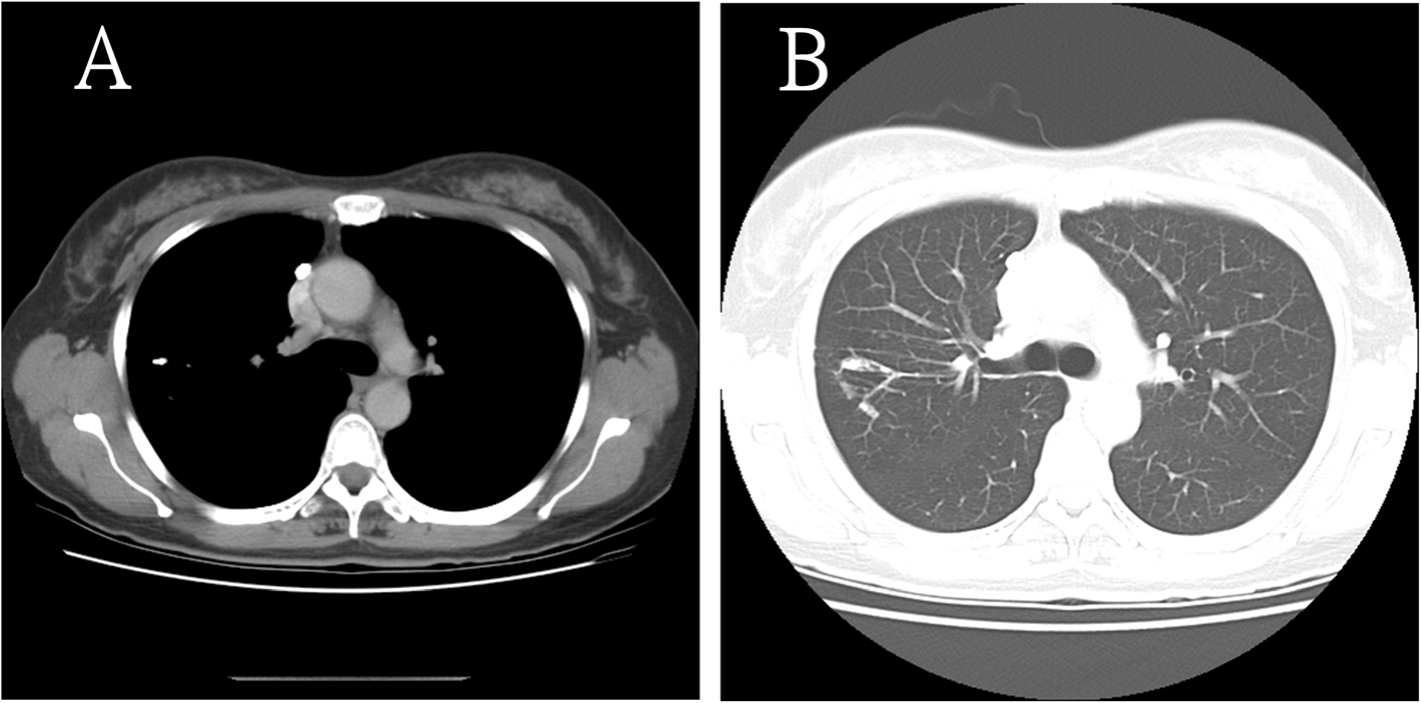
Initial chest computed tomography. Initial chest computed tomography shows a calcified nodule in the upper right lobe in the mediastinal window (yellow arrowhead) (a). The nodule is encased within the dilated bronchus (yellow arrowheads), with parenchymal infiltration in the lung window (blue arrowheads) (b)
